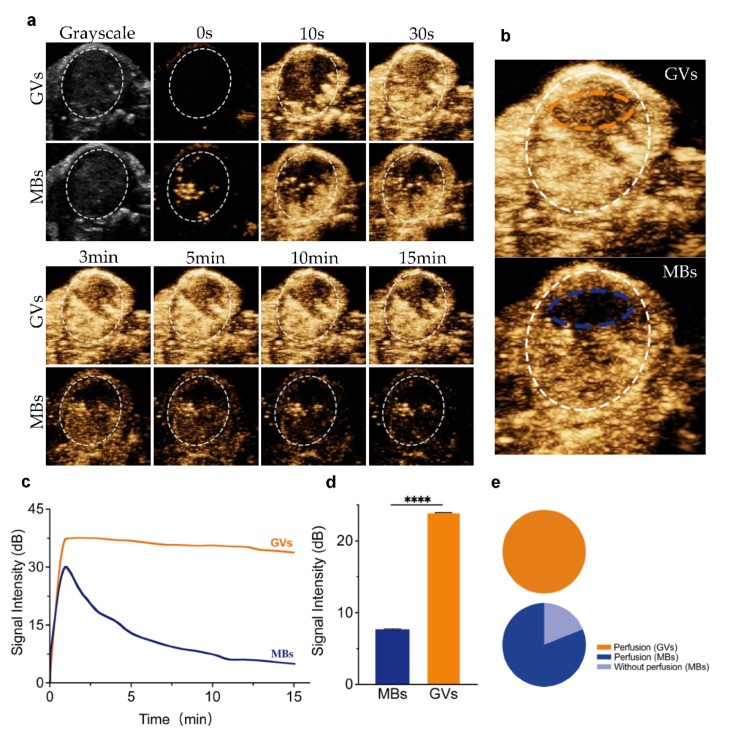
Ultrasound imaging of GVs and lipid MBs in tumor: (a) B-mode and nonlinear contrast images of GVs and MBs in 15 min after tail injection; (b) nonlinear contrast images of the ischemic zone of a tumor after injection of GVs (orange-dotted circle) or MBs (blue-dotted circle); (c) time–intensity curve of GVs and MBs perfused into the tumor tissue; (d) contrast signal intensities of GVs and MBs in the tumor’s ischemic zone (orange- and blue-dotted area); (e) the perfusion area of GVs and MBs in the tumor. Error bars represent ± SD.  *p* < 0.0001.
